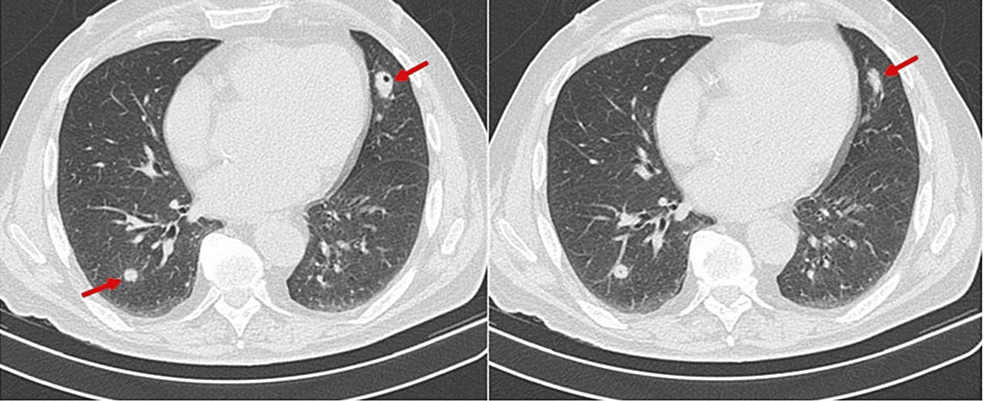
(Left, right) CT scan of chest Red arrows pointing toward multiple lung nodules of various sizes in bilateral lung fields
